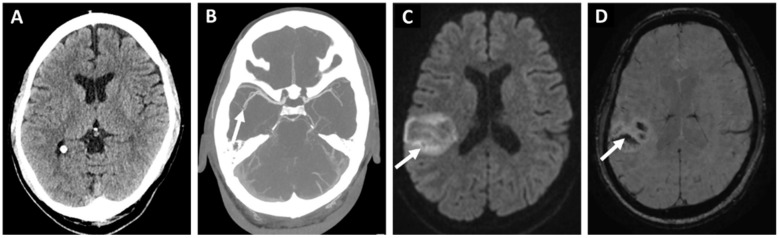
76-year-old woman with hypertension and dyslipidemia presented with a sudden onset left hemiparesis and dysarthria. Initial non-contrast computed tomography (A) showed no acute hypodensity. The patient received immediate intravenous thrombolysis after which computed tomography angiography (B) revealed a right-sided middle-cerebral artery occlusion in the M2 segment too distal to be treated with endovascular treatment. Despite thrombolysis, the next day magnetic resonance diffusion-weighted imaging (C) showed a cortical infarction (arrow) with a moderate hemorrhagic transformation in the susceptibility weighted imaging (D). Etiologic classification for the stroke was embolic stroke of undetermined source (arrow) after initial diagnostic work-up. And implantable loop monitor inserted 11 weeks later revealed a paroxysmal atrial fibrillation as the probable source of the embolism.
